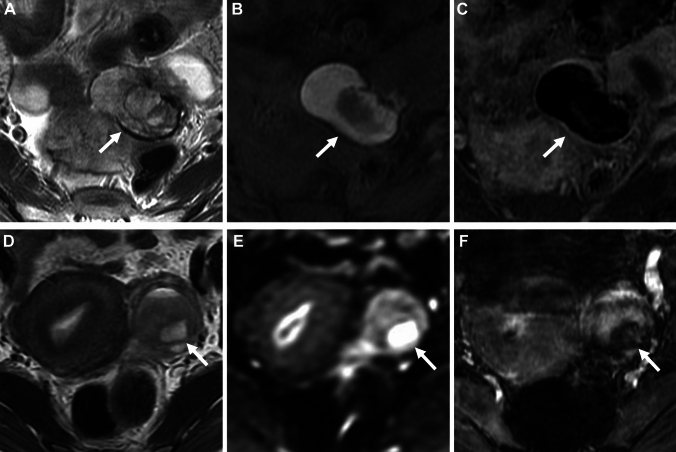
Clots in endometrioma. A 34-year-old with suspicion of an ovarian cystic mass with mural nodules on ultrasonography examination. A T2-weighted image, B fat-saturated T1-weighted image, and C contrast-enhanced subtraction image show a left endometrioma (arrow) with a solid component. The solid component shows intermediate signal intensity on T2-weighted image. The high signal intensity of cyst contents masks the signal of the solid component on the post-contrast image, and no contrast enhancement of the clot is revealed on the contrast-enhanced subtraction image. A 27-year-old with a history of dysmenorrhea. D T2-weighted image, and E DWI (*b* = 800 s/mm^2^) show a left endometrioma (arrow) with a solid component. The solid component shows intermediate signal intensity on T2-weighted image and water diffusion restriction on DWI, however, appears as a signal void on F SWAN suggesting a clot
